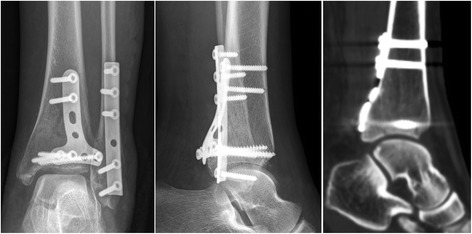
For the patient presented in Fig. 4, a distal radius plate was applied spanning the fracture in a buttress mode to fix the PMF. The postoperative sagittal reconstruction of CT images confirmed an excellent (anatomical) reduction of the posterior fragment
